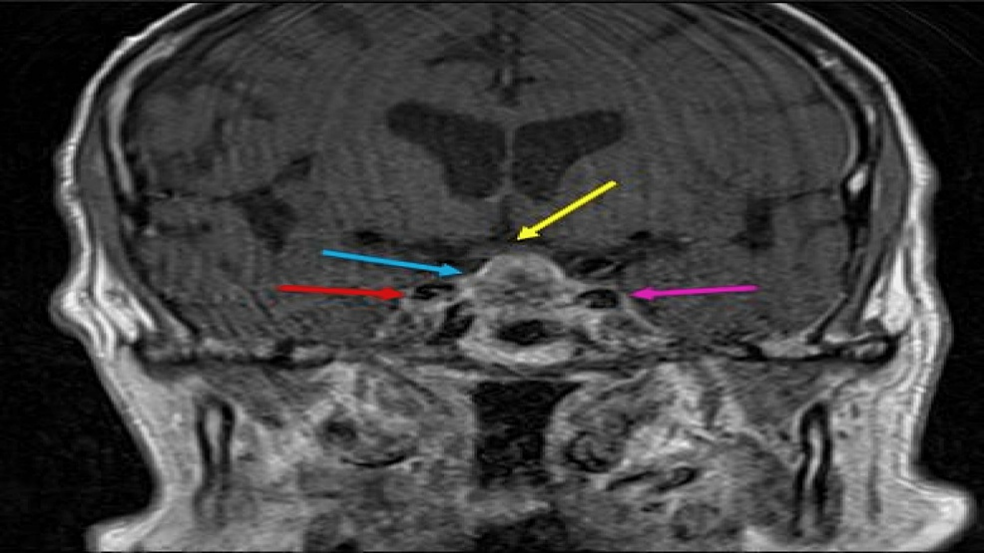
Coronal T1 post-contrast MRI of the pituitary The pituitary mass shows heterogenous enhancement (blue arrow). There is the involvement of the cavernous sinuses (more on the left) with internal carotid arteries along the right side (red arrow) and left side (pink arrow). The suprasellar extension is contacting the undersurface of the optic chiasm (yellow arrow) with no overt compression.
